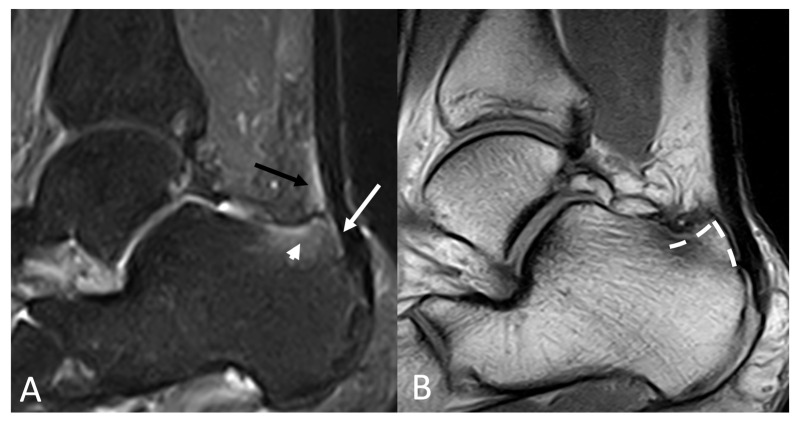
(A) Sagittal STIR and (B) sagittal T1-weighted of a 37-year-old male with pain and a bump at the level of calcaneal insertion of the Achille’s tendon. Bony edema of the calcaneus (arrowhead) and the area of impingement between the bone and the tendon (arrow). Retrocalcaneal bursitis is evidenced by increased fluid and inflammation (black arrow). A bony bump on the posterosuperior calcaneus (dashed line) is present, consistent with Haglund’s deformity.
